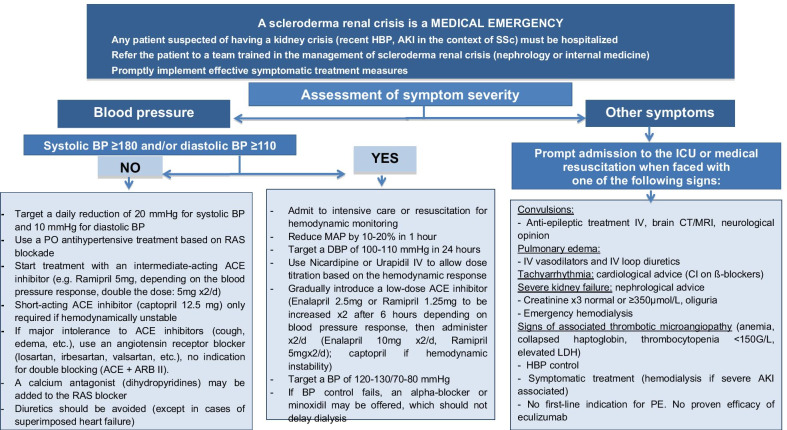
Management of scleroderma renal crisis. *BP* blood pressure, *PO* per os, *RAS* renin–angiotensin system, *ACE* conversion enzyme inhibitors, *ARB II* angiotensin receptor blockers II, *MAP* mean arterial pressure, *IV* intravenous, *CT* computerized tomography, *MRI* magnetic resonance imaging, *CI* contraindication, *IV* intravenous, *HBP* high blood pressure, *AKI* acute kidney injury, *PE* plasma exchange, *LDH* lactate dehydrogenase
